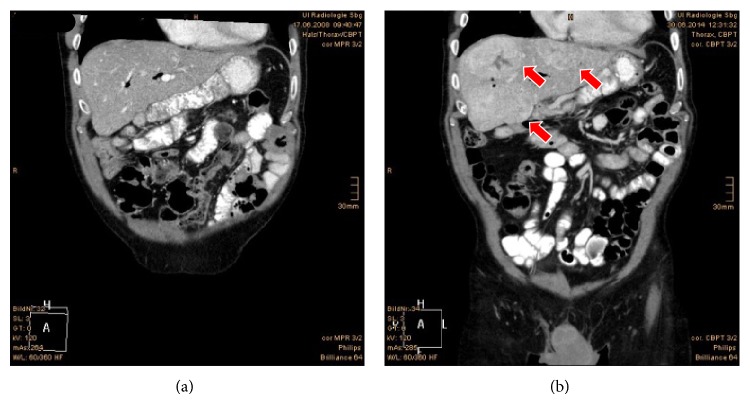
(a) Abdomen CT coronal view showing no evidence of metastasis after multiple metastasectomies. The scan was performed in 2008. (b) A recent (2014) picture of the same view showing multiple liver metastases (red arrows) before starting the 8th systemic targeted therapy.
